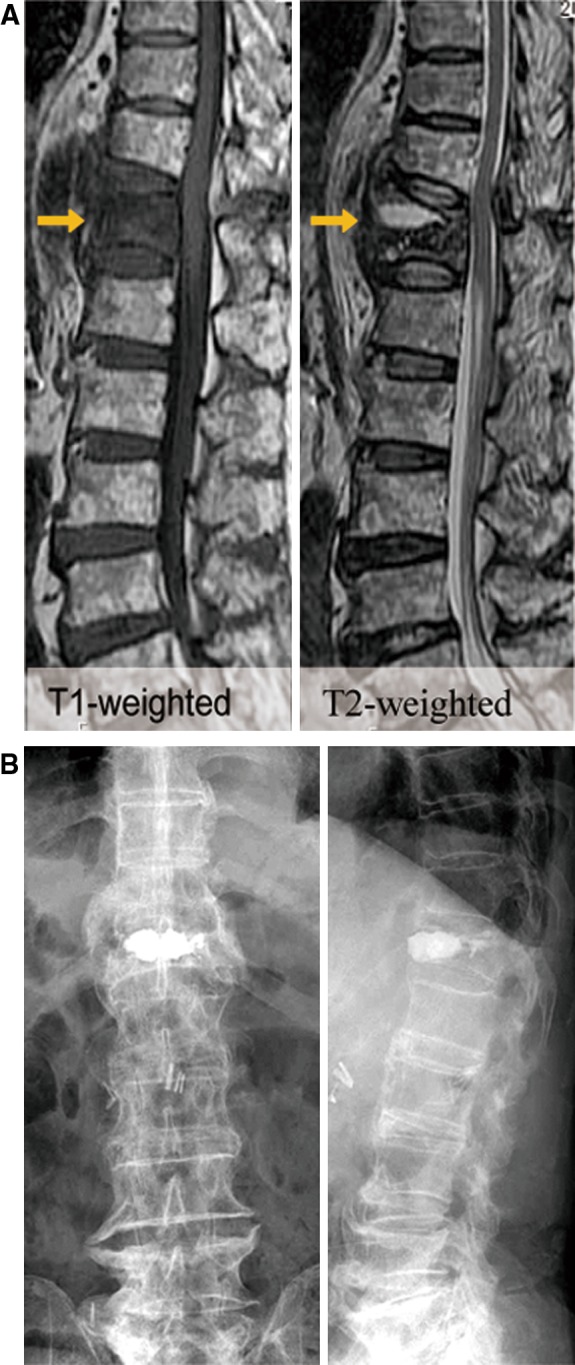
(Case 2) a Sagittal T1-andT2-weighted images showing collapsed vertebral body with formation of a cavitary lesion at the L1 level. b Radiograph of the spine after BKP reveals effective filling of cystic fracture cavity
